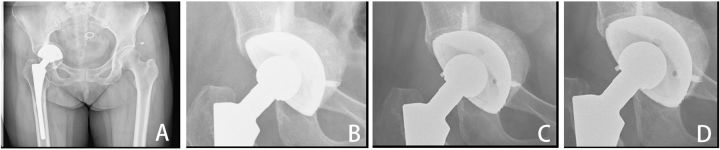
A 58-year-old woman developed prosthetic cup loosening 4 years after THA. Pre-operative radiograph showed massive acetabular bone defect (A). MC grafts were used to reconstruct the acetabulum and radiographs taken at 3rd month (B), 6th month (C) and final follow-up (D) showed the bone grafts were well remodeled.
